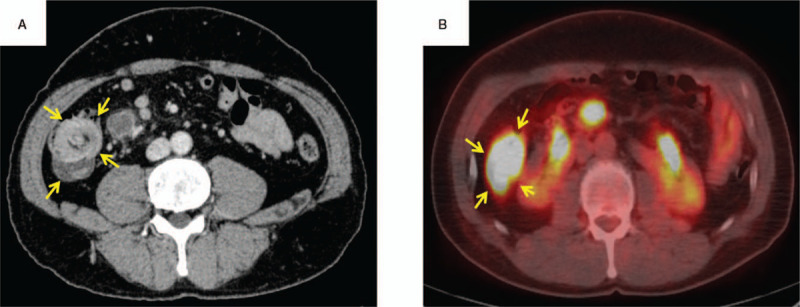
A, PET image and B, contrast-enhanced CT image of the coronal section, January 2017. CT = computed tomography; PET = positron emission tomography.
